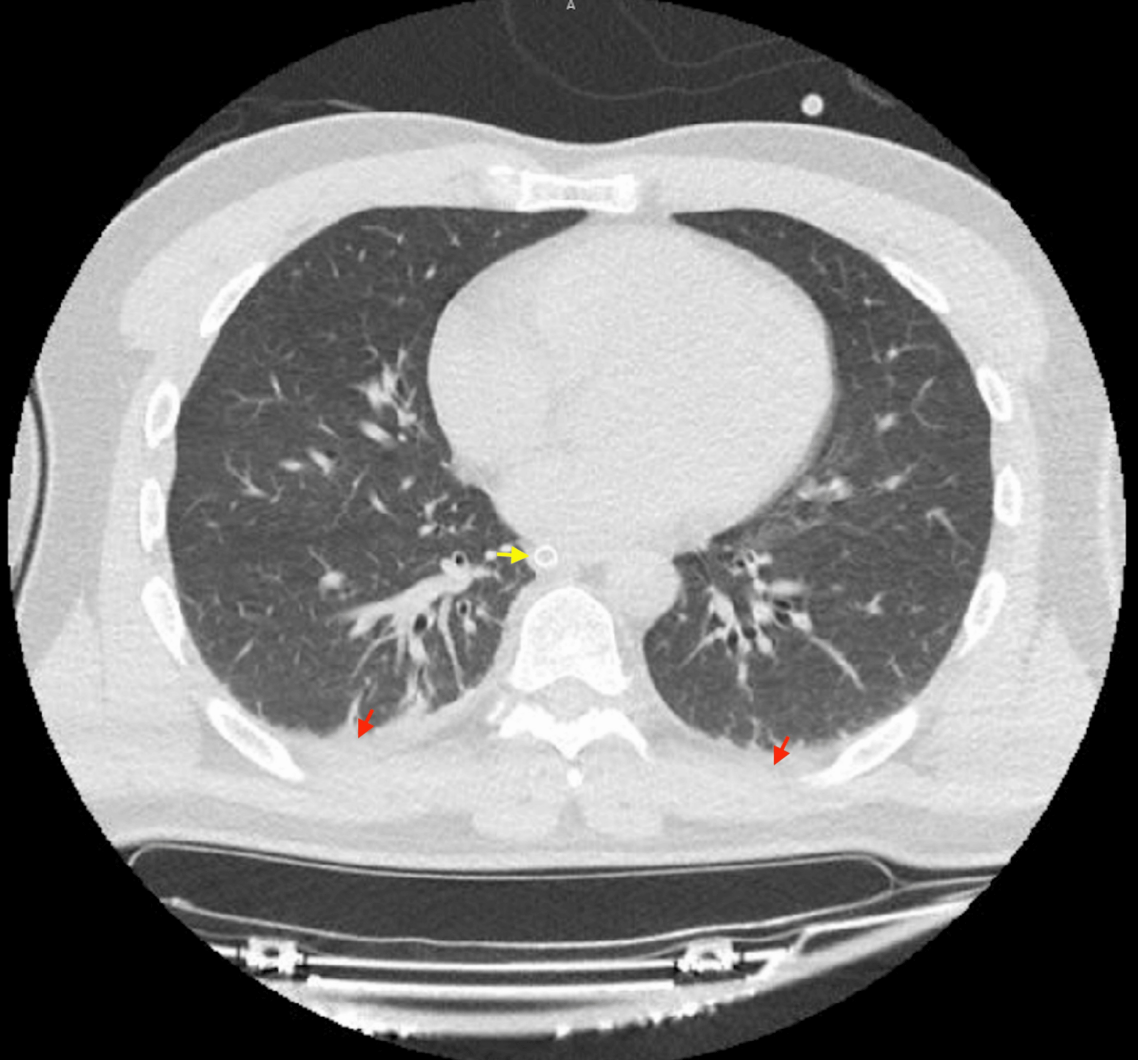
Chest CT scan. Volumetric acquisition using multi-detector equipment with thin slices and high-resolution images. No contrast medium was administered. The red arrow shows a small pleural effusion, and the yellow one indicates the orotracheal tube. Apart from these signs and a right clavicle fracture sequela (not visible in this figure), the official report indicated no abnormalities.
